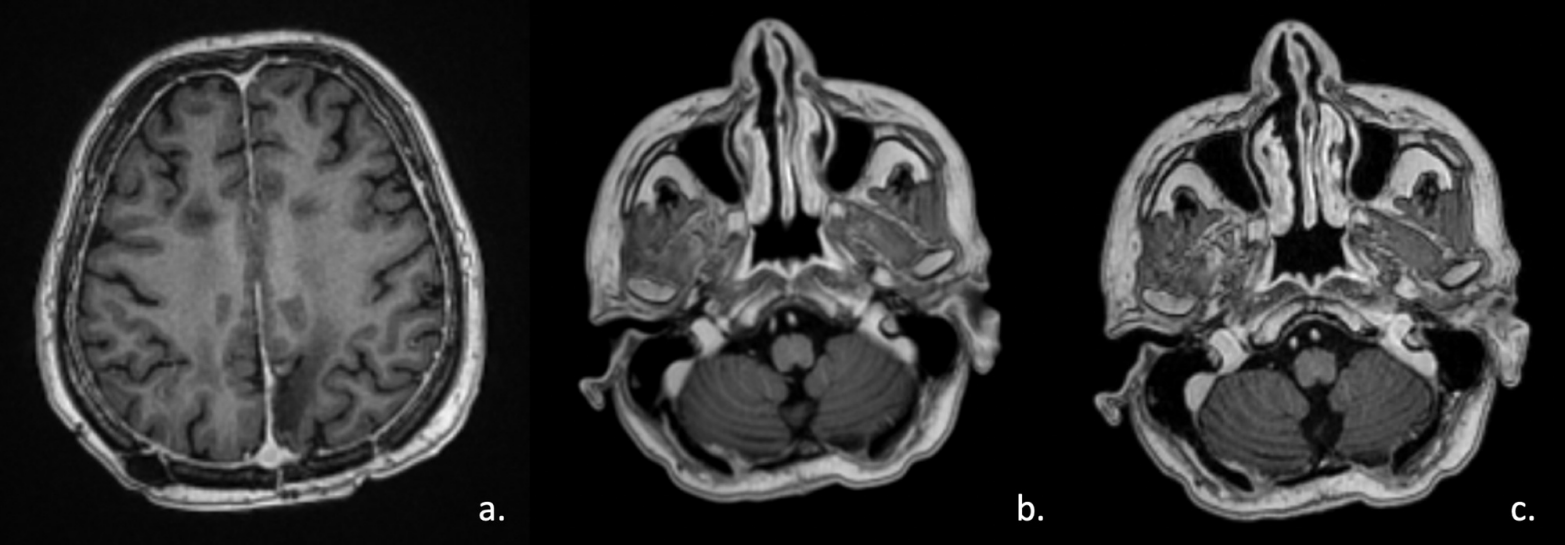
Axial contrast-enhanced MRI scans of the lesions a. In the left parietal lobe. b. In the right cerebellar hemisphere. c. In the left cerebellar hemisphere one year after the stereotactic radiosurgery treatment.
